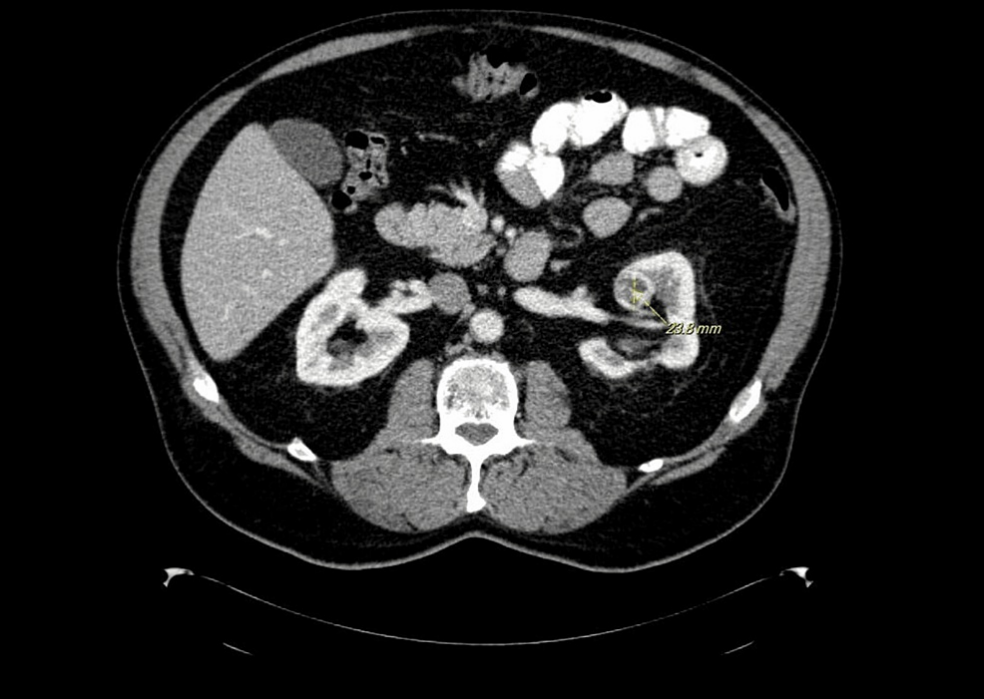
CT-scan revealed a mixed solid and cystic mass lesion on the superior pole of the left kidney.
